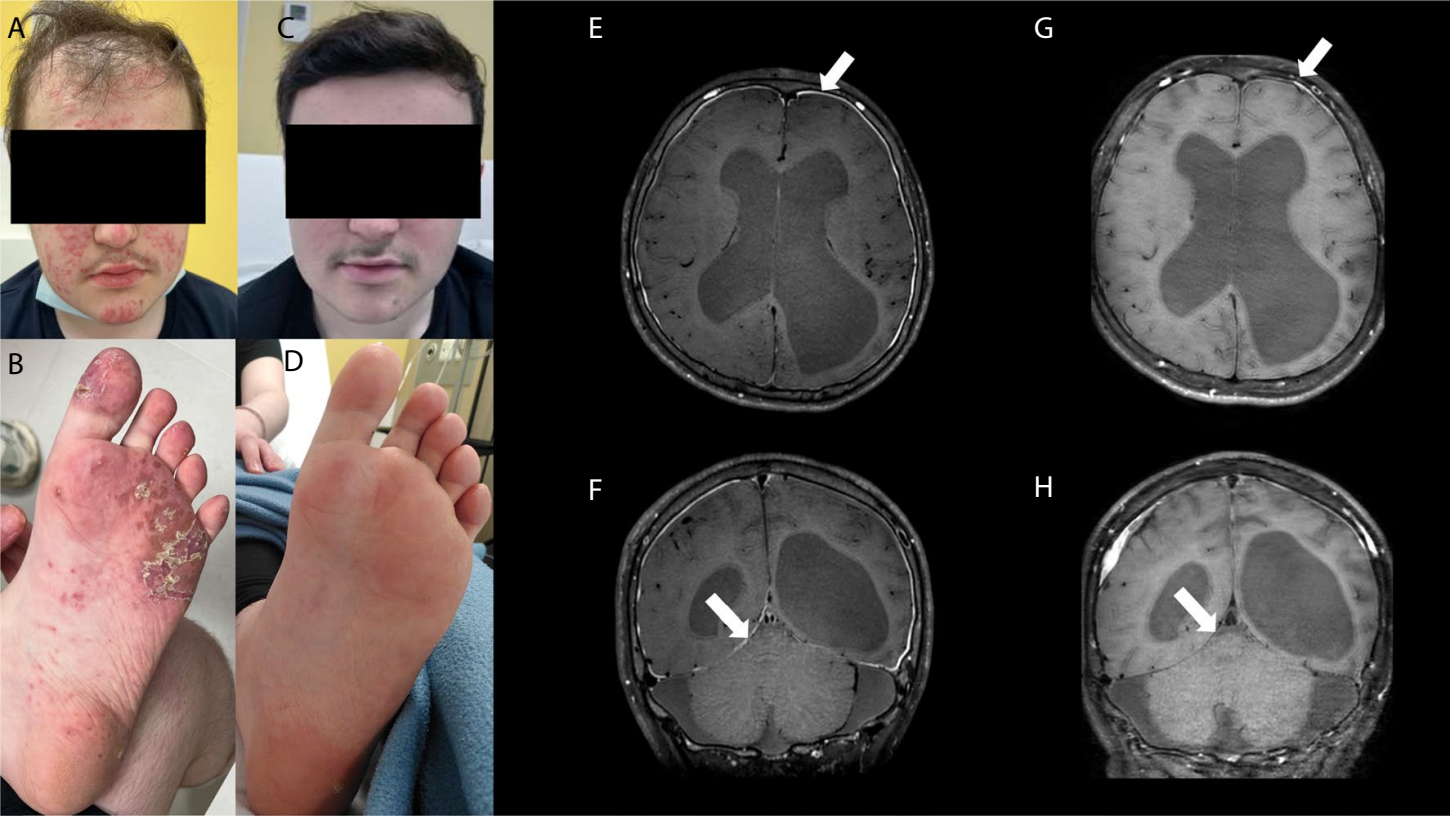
Clinical and radiological effects of JAKi in a patient with C1QDef. A-D Clinical picture of the face of patient AGS3489 and foot 2 weeks before (A-B) and 9 months after (C-D) initiation of treatment with baricitinib. The complete list of therapies at each time point is given in Supplementary Table 2. E–F Cerebral MRI (T1 sequence with contrasts) of patient AGS3489 3 months before initiation of baricitinib showing cerebral atrophy and pachymeningitis (arrows indicate meningeal thickening with enhancement). G-H Evolution of MRI after 6 months of baricitinib showing improvement in meningeal thickening and enhancement. Of note, a subdural hematoma appeared after lumbar puncture
